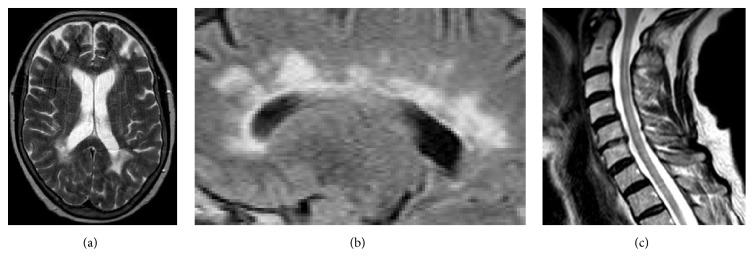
Brain and spinal MRI of the patient following admission on 12 October 2014. (a) Brain T2W axial MRI (1.5 T) demonstrating the characteristic periventricular lesions of MS. (b) Magnification of brain FLAIR sagittal MRI showing involvement of the corpus callosum. (c) Sagittal T2W spinal MRI of the cervical cord with diffuse, patchy lesions. T2W: T-2 weighted; FLAIR: Fluid-Attenuated Inversion Recovery; T: Tesla.
